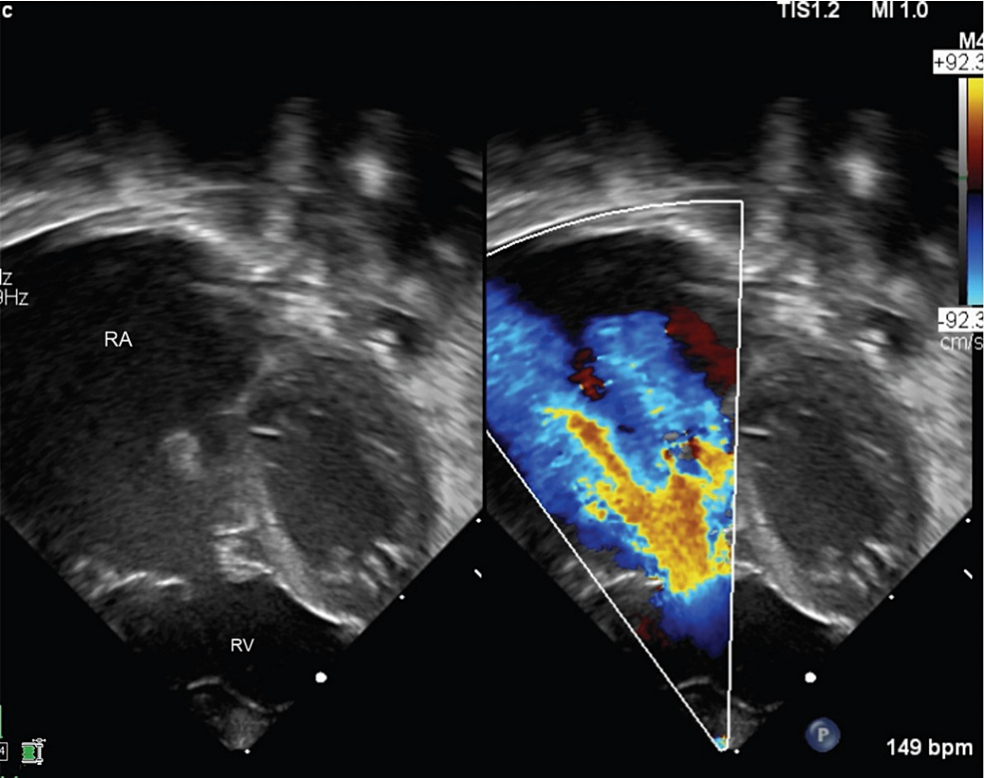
Neonatal echocardiogram showing apical four-chamber view of Twin A with apically displaced tricuspid valve, RA dilation, and severe tricuspid regurgitation RA, right atrium; RV, right ventricle
